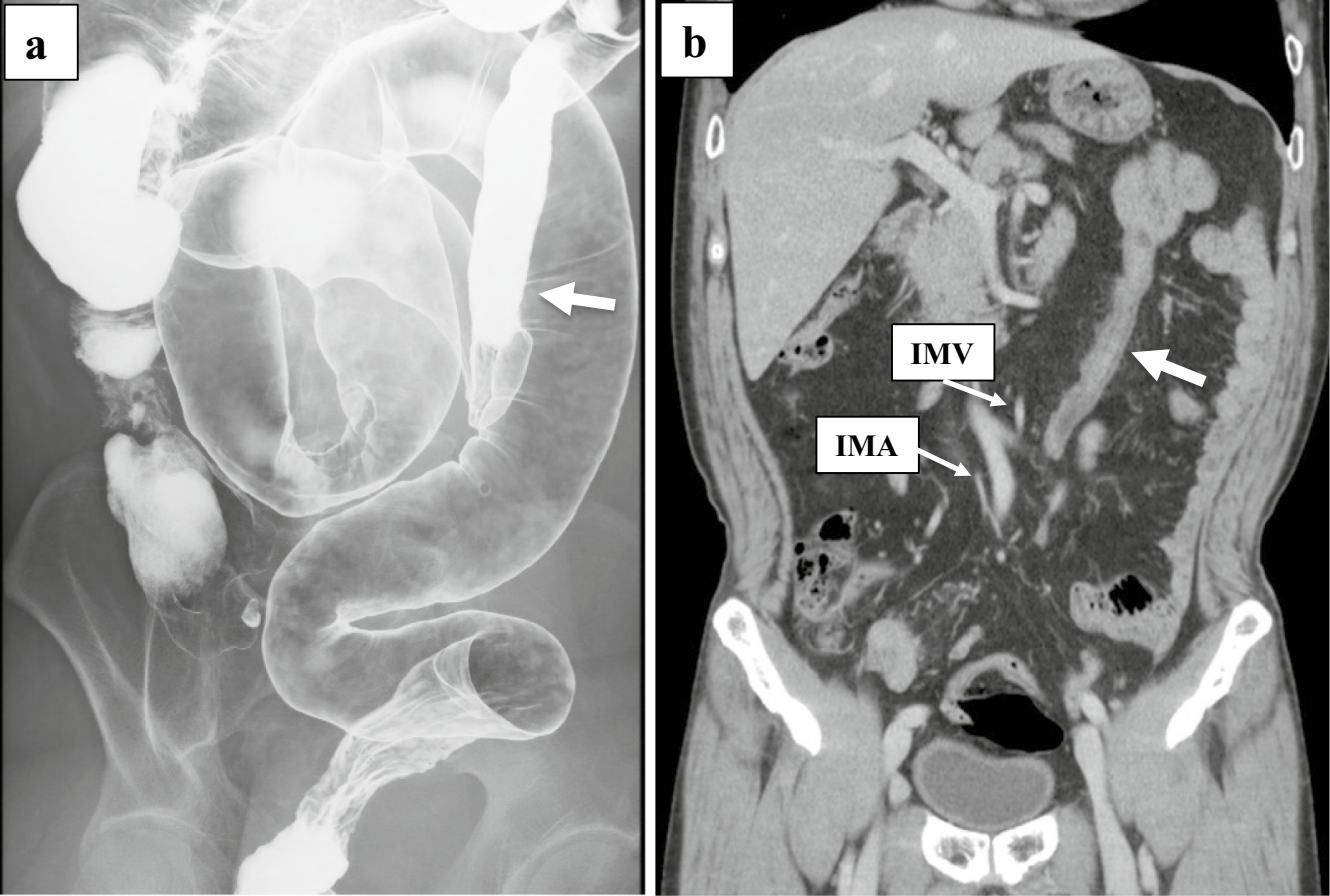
Case 2. a BE revealed moderate displacement of the descending colon to the midline (white arrow) and the sigmoid colon was quite long. b In an enhanced CT, the white arrow showed the descending colon. IMA, IMV, and the descending colon ran very close to each other
